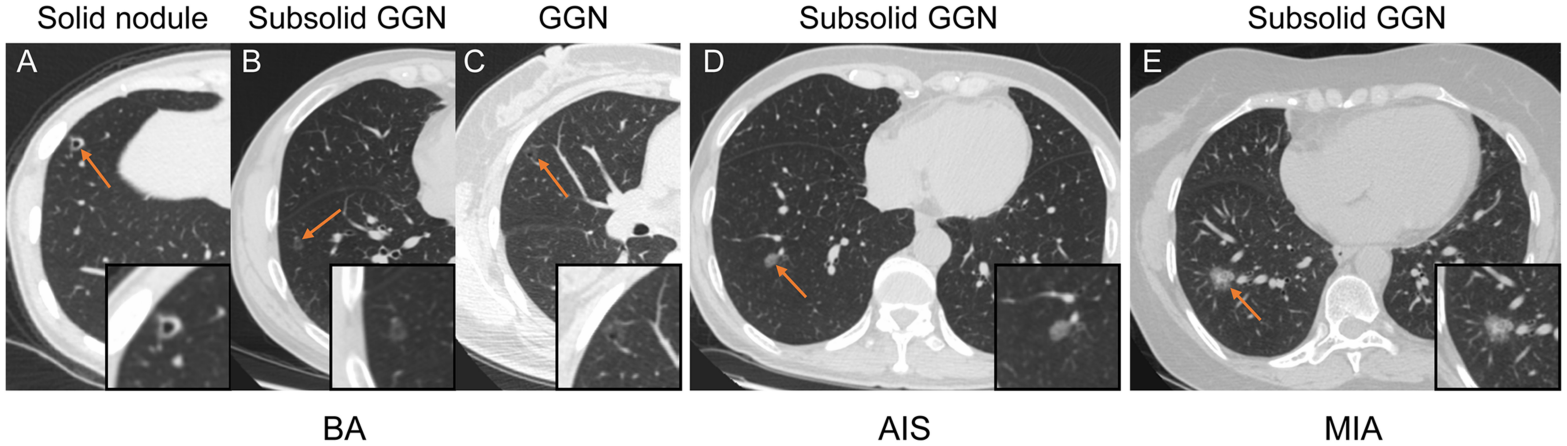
Representative CT images of patients with BA (A–C), AIS (D), and MIA (E). (A–C) Axial CT image of BA presenting as a solid nodule (A), subsolid GGN (B), and GGN (C) with round to oval shape, pseudo-cavitation, smooth margins, and clear tumor–lung interface. (D) Axial CT image of AIS that presents as a subsolid GGN with an oval shape, smooth margins, and clear tumor–lung interface. (E) Axial CT image of MIA appearing as a subsolid GGN with an oval shape, small spiculated margin, shallow lobulation, and clear tumor–lung interface.
